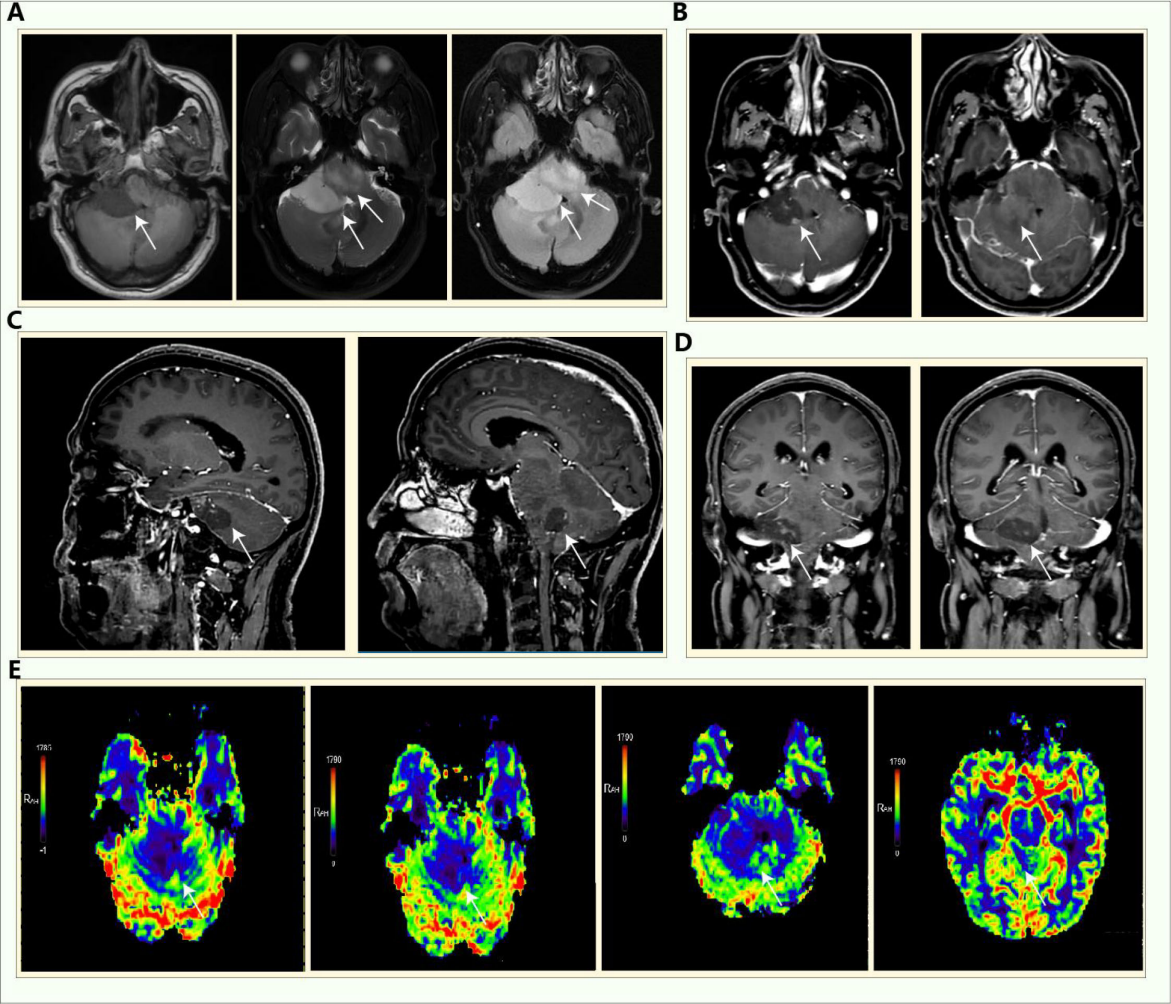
Multimodal enhanced MRI for brain tumor of the patient before the operation. (A) The T1, T2 and FLAIR sequences respectively; (B-D) the axial position, sagittal position and coronal position of the enhanced scan; (E) the perfusion imaging of the patient.
